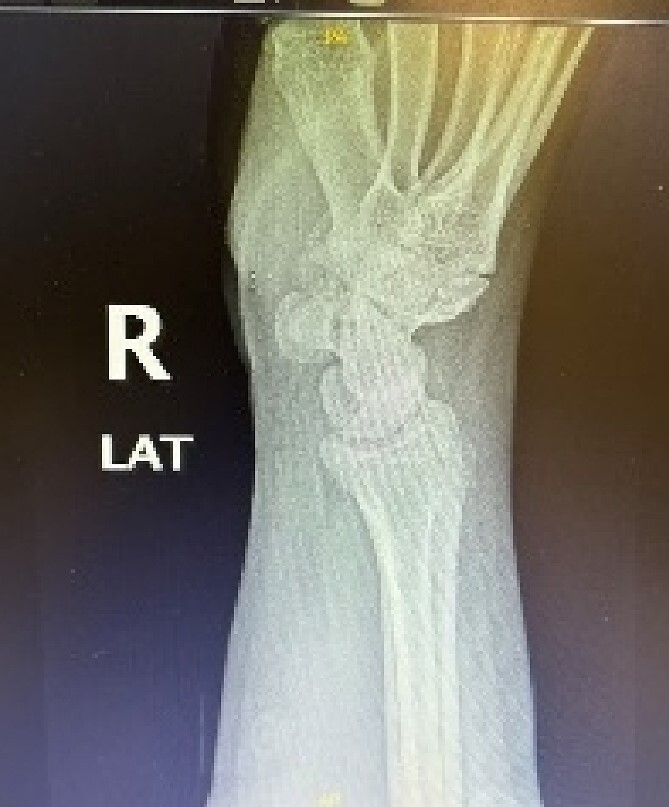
Hand and wrist x-rays pre-operatively.
